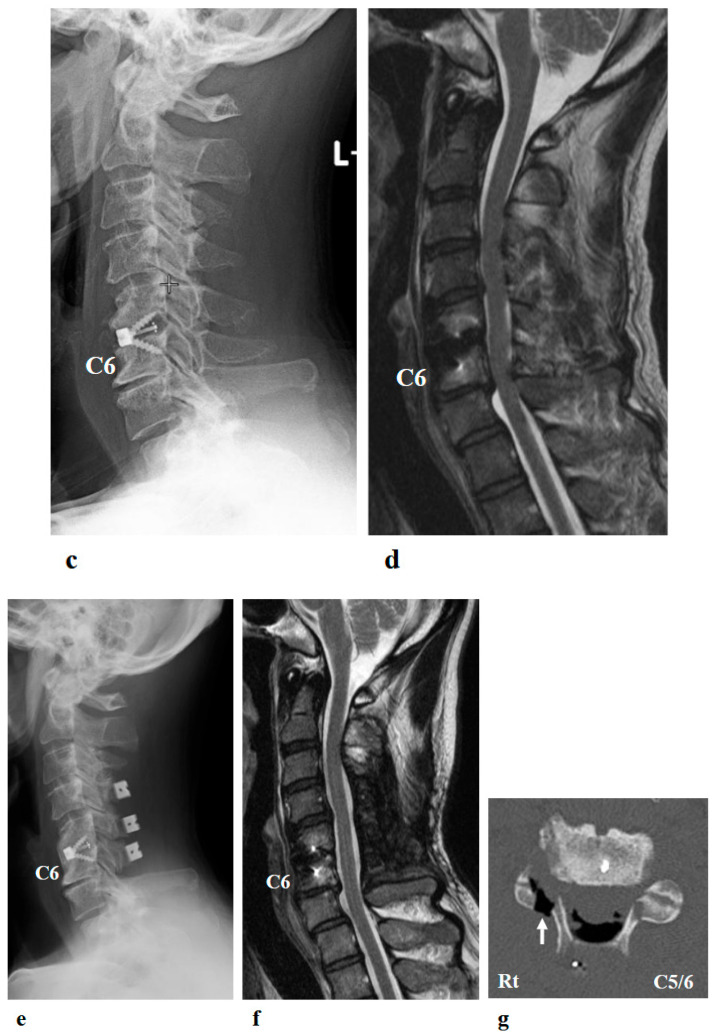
Preoperative (a,b) and postoperative (c,d) images following the first surgery, as well as postoperative images from the second surgery (e–g). (a) Lateral cervical spine X-ray showing narrowing of the intervertebral disc space and osteophyte formation at C5–C6 and C6–C7. (b) Reconstructed mid-sagittal CT image after myelography revealing spinal cord compression at C5–C6 and C6–C7, and posterior displacement of the C5 vertebral body. (c) Postoperative lateral cervical spine X-ray after anterior decompression and fusion at C5–C6. (d) Mid-sagittal cervical MRI demonstrating persistent spinal cord compression from C3–C4 to C6–C7. (e) Postoperative lateral cervical spine X-ray after C4–C6 laminoplasty and partial laminectomy at the caudal aspect of C3 and the cranial aspect of C7. (f) Mid-sagittal cervical MRI showing adequate spinal cord decompression from C3–C4 to C6–C7. (g) Axial CT image at the C5–C6 level showing adequate right C5–C6 foraminotomy (arrow).
